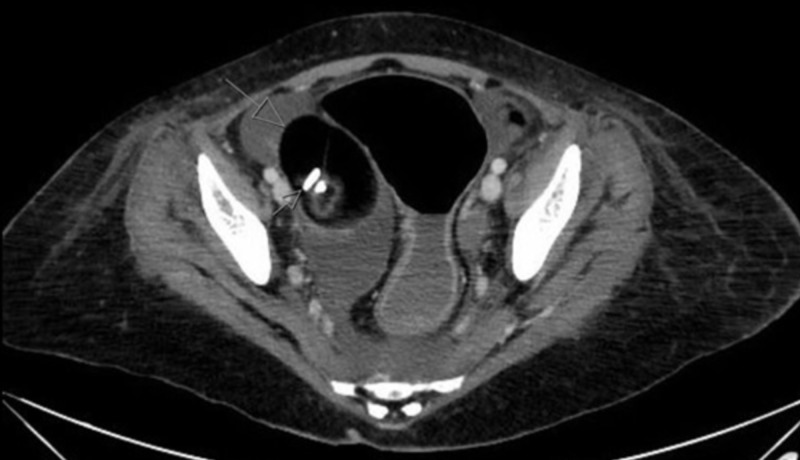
Preoperative CT scan of the abdomen/pelvis Cross-sectional computed tomography (CT) scan of abdomen/pelvis showing a 7.2 x 6.3 x 5.5 cm mass of the right ovary that was highly suspicious for a mature teratoma with fat densities and calcified foci
